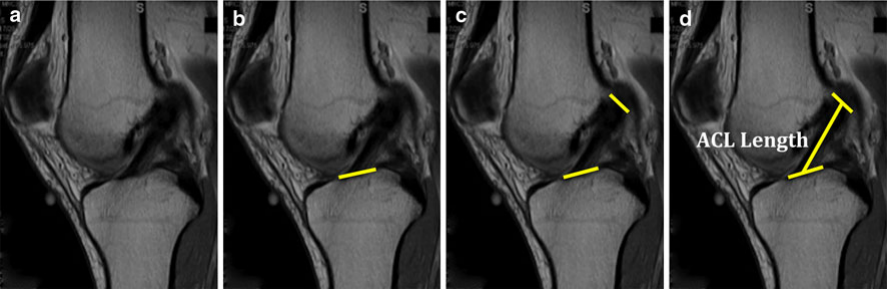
a MRI sagittal cut best showing tibial and femoral insertion sites is chosen; b tibial insertion site is highlighted; c femoral inserion site is highlighted; d the distance between the mid-portion of the tibial and femoral insertion sites are connected and measured to know ACL length
